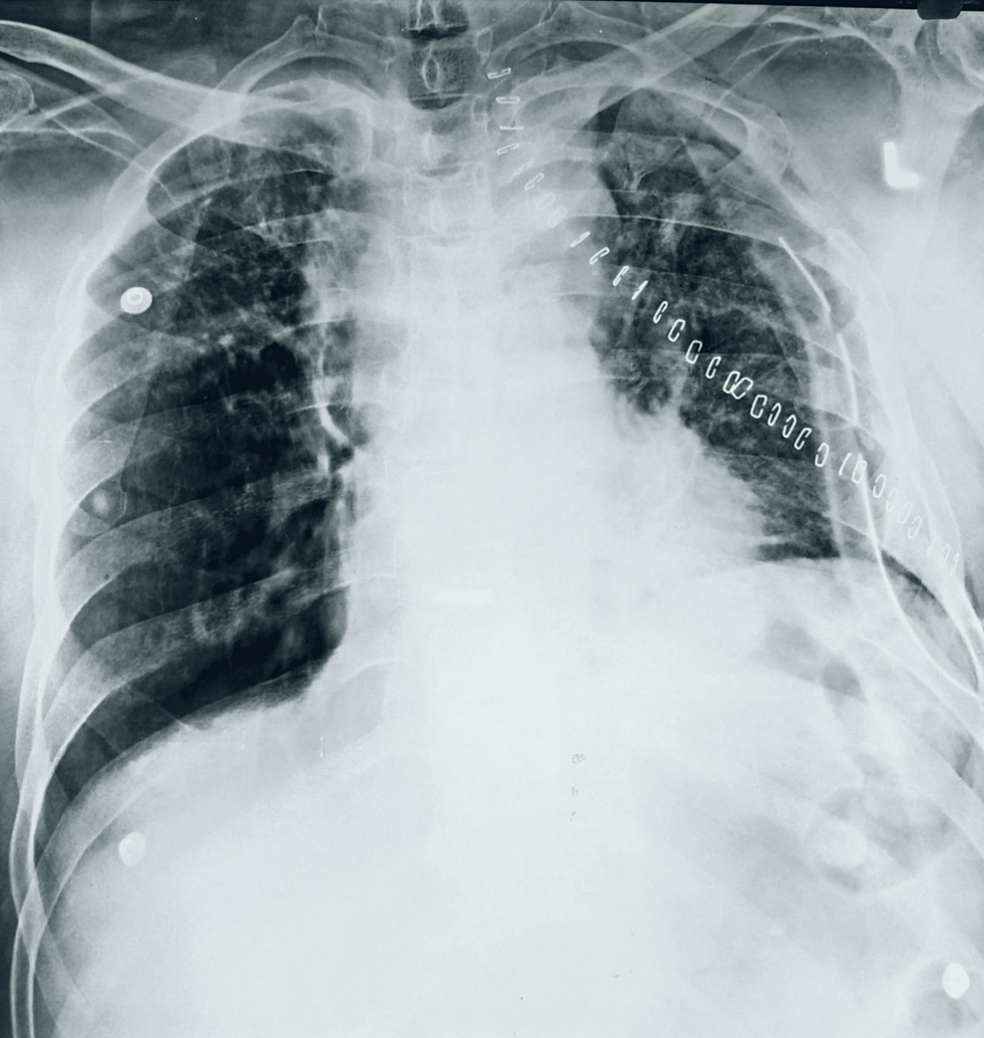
Post-operative chest radiograph Chest X-ray showing adequate left lung expansion following upper lobectomy.
